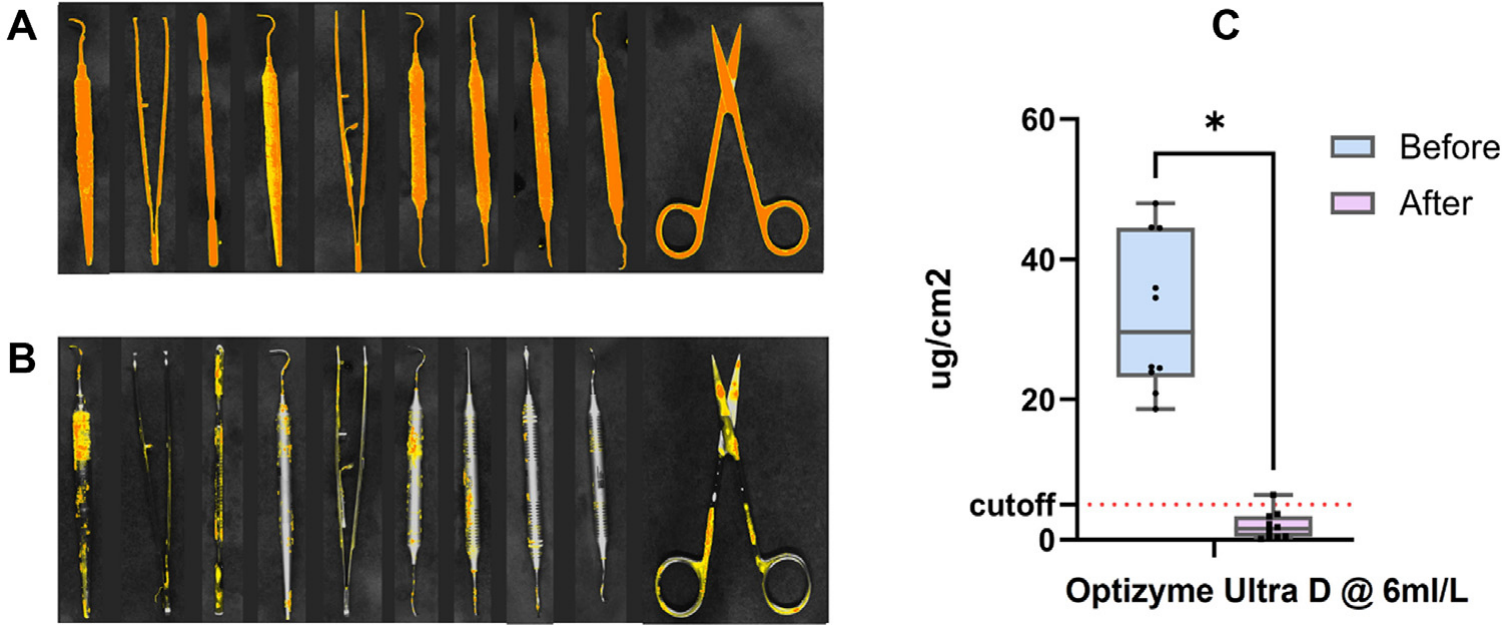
Visual and quantitative analysis of residues on artificially soiled dental instruments using PROReveal for a long ultrasonic cleaning cycle of 30 minutes using product M. The instruments were dried for 3 days before cleaning. Panel (A) shows the instruments immediately before cleaning; while panel (B) shows the instruments after cleaning. Panel (C) compares the levels before and after.
